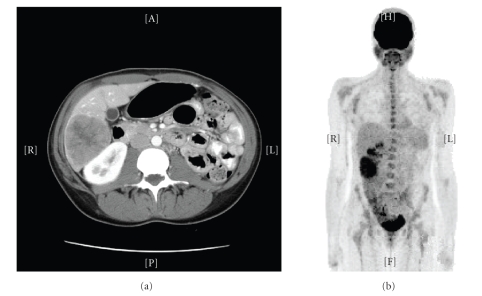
CT and PET scans of EBV-related SMN of liver. CT scan of the abdomen and pelvis (8/05) from Patient 3 revealed multiple masses, mainly in the right lobe and involving segment 4. The largest mass measured 6.8 × 5.8 cm and was located in the right inferior lobe (a). FDG-PET (9/05) demonstrated areas of hypermetabolism in the inferior aspect of the right hepatic lobe (SUV max 5.7), and two other neighboring foci (SUV max 5) (b).
